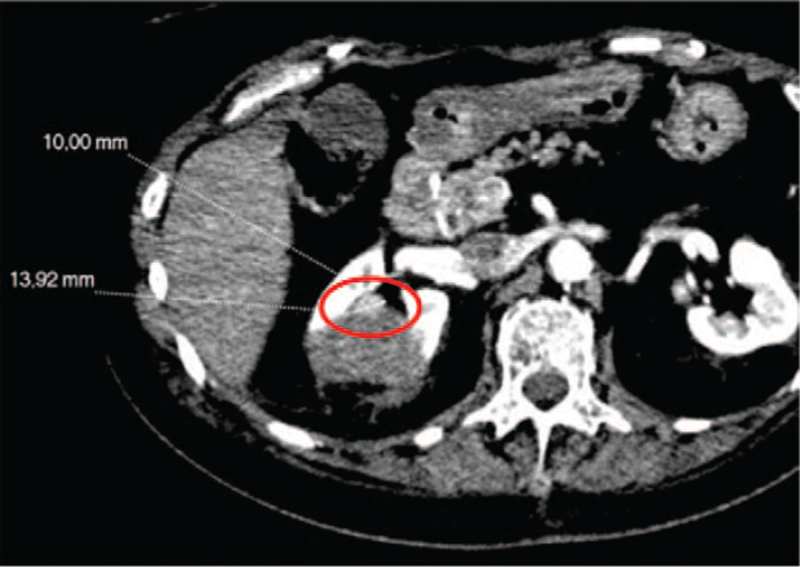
Abdomen CT scan post cryoablation: the red circle identifies the residual disease. Please, note the intratumoral arterial enhancement near to renal pelvis.
